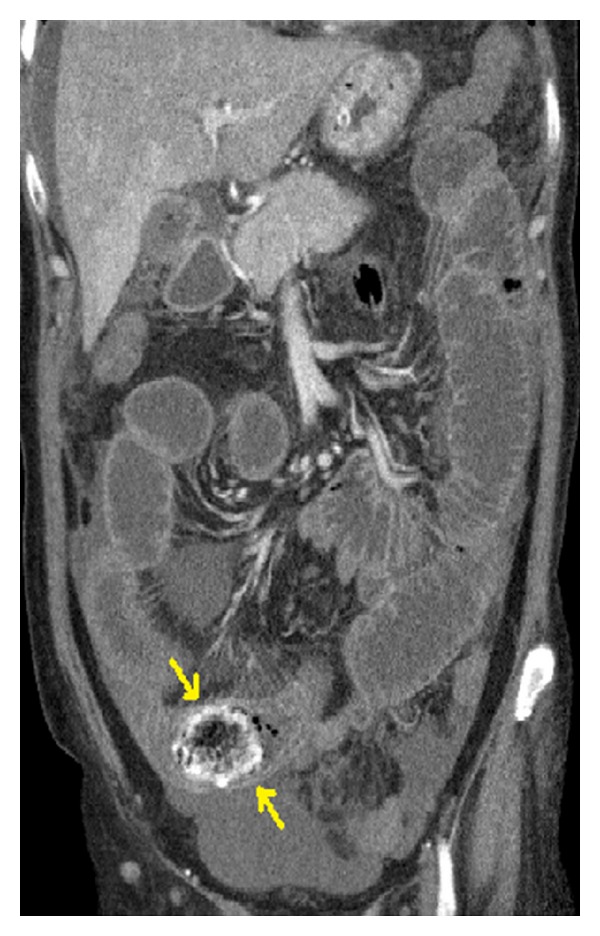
Obstructive lesion in terminal ileum and proximal dilatation on abdominal CT.
